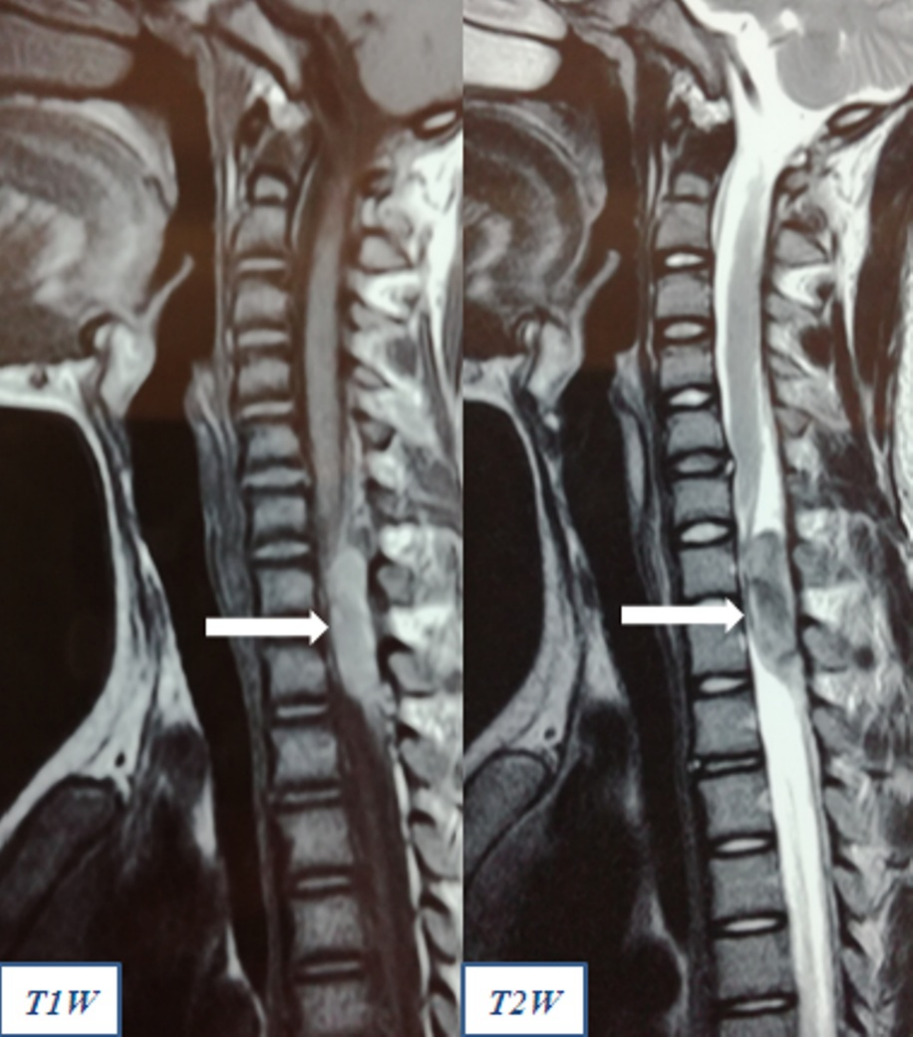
Magnetic resonance images suggestive of an extra-dural hematoma in the cervico-thoracic junction
